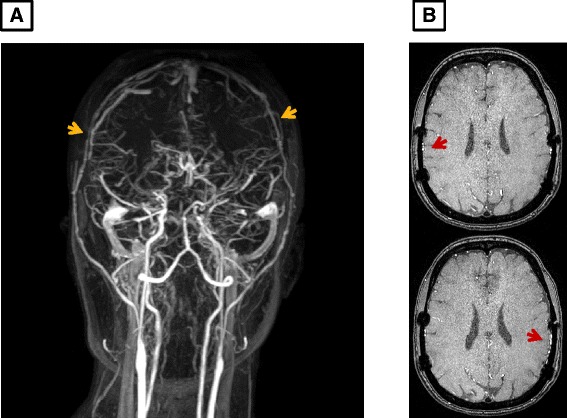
Brain magnetic resonance imaging and angiography after indirect revascularization (arrows). A: Magnetic resonance angiography, coronal view; B: axial view.
